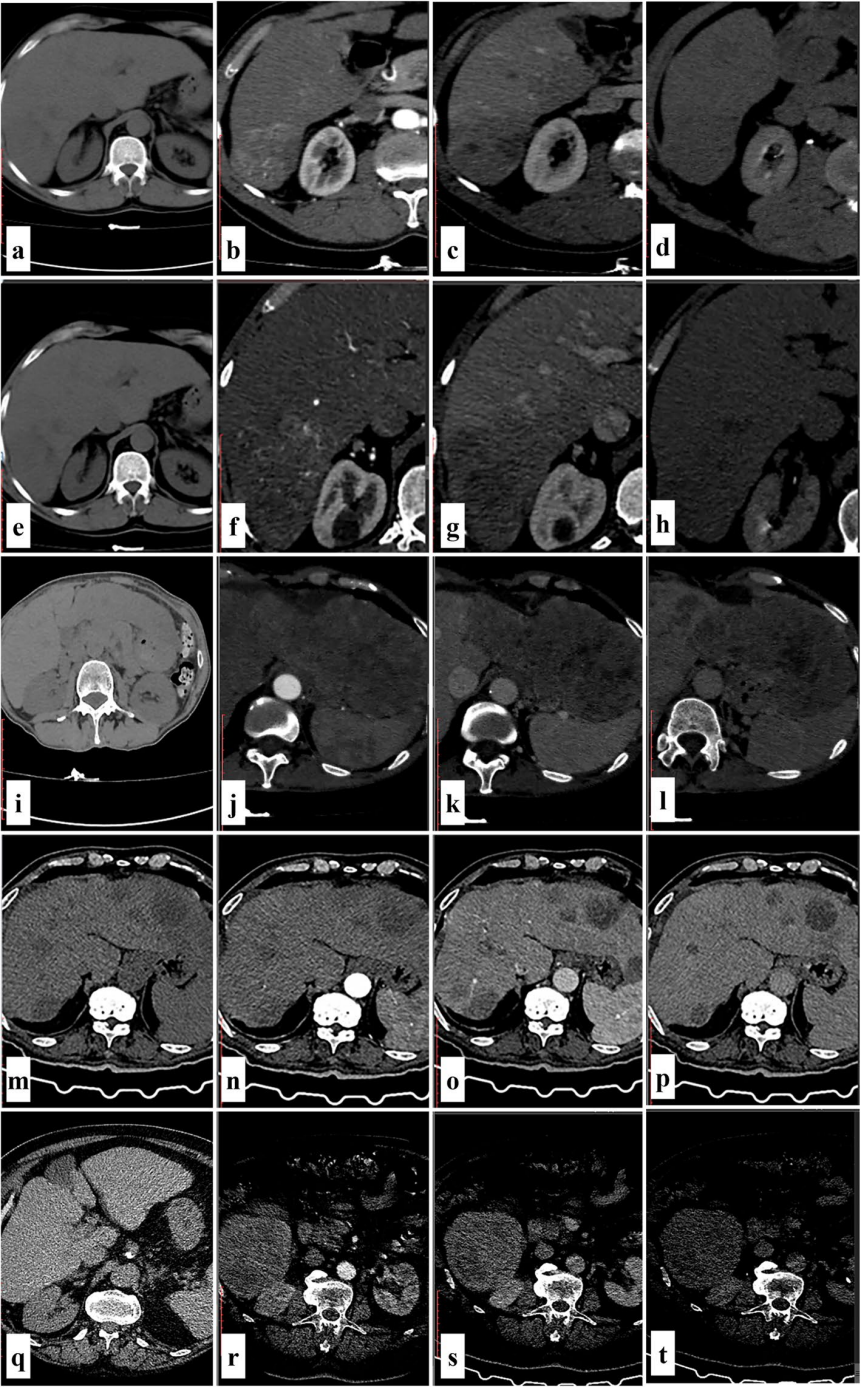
Contrast-enhanced triphasic CT imaging of typical and atypical HCC cases: A case of typical radiological appearance of multiple HCC (LR-5) (a–d). a Cirrhotic liver changes, b with right hepatic lobe focal lesion seen at segment VI displaying intense arterial enhancement, c with washout of contrast in portovenous phase, d being hypo dense to hepatic parenchyma in delayed equilibrium study. e–h Another focal lesion is seen at segment VII with similar enhancement pattern. A case of infiltrative HCC (LR-5) (i–l). i Cirrhotic liver changes, j with malignant infiltration of the left hepatic lobe that shows heterogeneous enhancement in the arterial phase with low density areas indicative of necrosis k and displays wash out of contrast at portovenous phase, l being hypodense to hepatic parenchyma in delayed equilibrium study. A case of multiple hypovascular HCC (LR-5) (m–p). m Cirrhotic liver changes, n–p with multiple bilobar variable sized hepatic focal lesions showing no contrast uptake in different study phases, the largest at left hepatic lobe segment II measuring 5 × 4.8 cm. A case of HCC on top of non-cirrhotic liver (LR-M) (q–t). q Non cirrhotic liver, r with right hepatic lobe segment VI large exophytic well defined focal mass lesion displaying thick irregular peripheral arterial enhancement and central hypo dense area of necrosis, s with washout of contrast at portovenous phase, t and delayed phases
